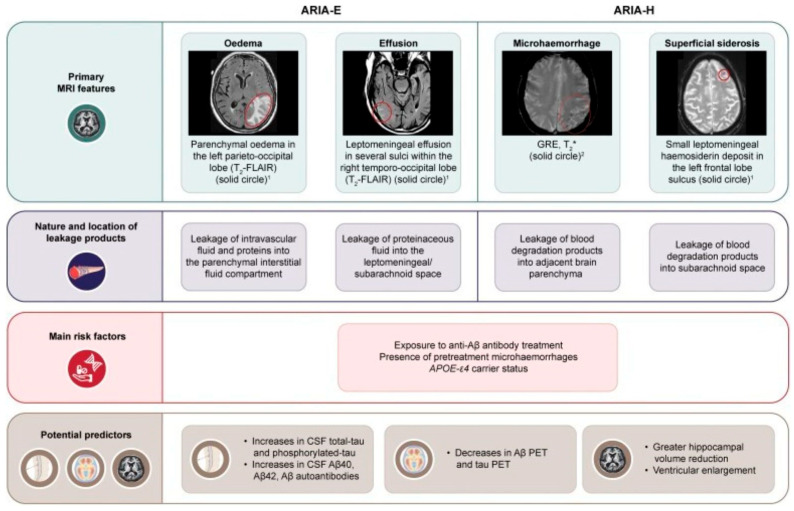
Characteristics of ARIA-H and ARIA-H, that include primary MRI features, nature, location, main risk factors, and potential predictors. Figure by Hampel et al. [14], licensed under CC BY 4.0.
